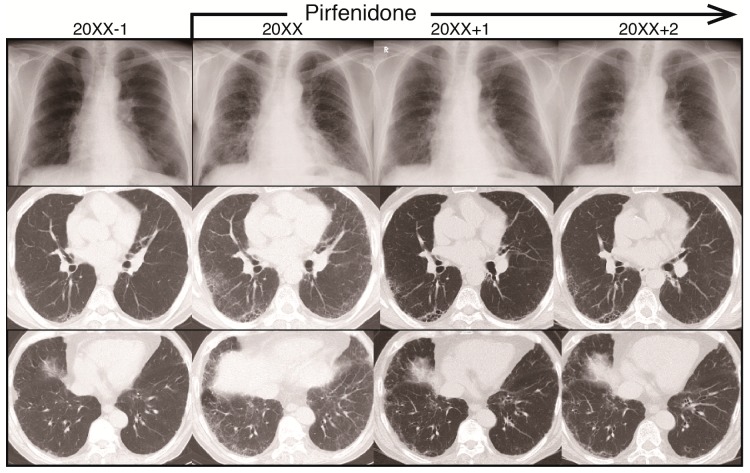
Radiograph images during the clinical course of idiopathic pulmonary fibrosis (IPF) for one year before the two years after initiation of pirfenidone treatment. Upper panel: reticular shadow on chest radiograph. Middle and lower panels: computed tomography (CT) images of influence of pirfenidone on IPF over a two-year period.
